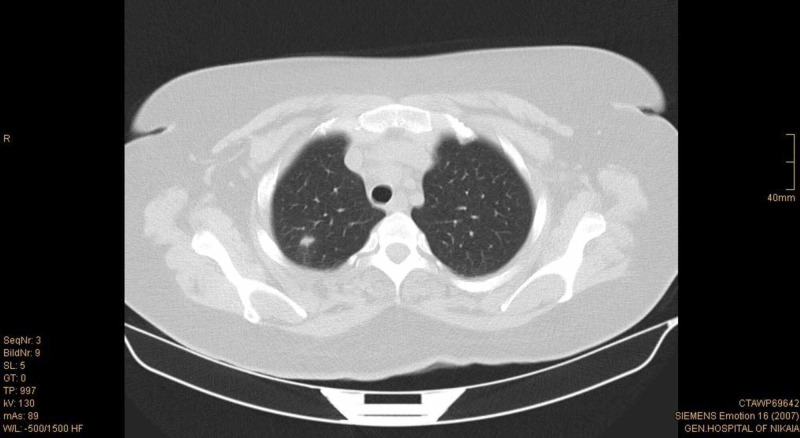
Chest CT scan that shows a nodule at the right upper pulmonary lobe.
